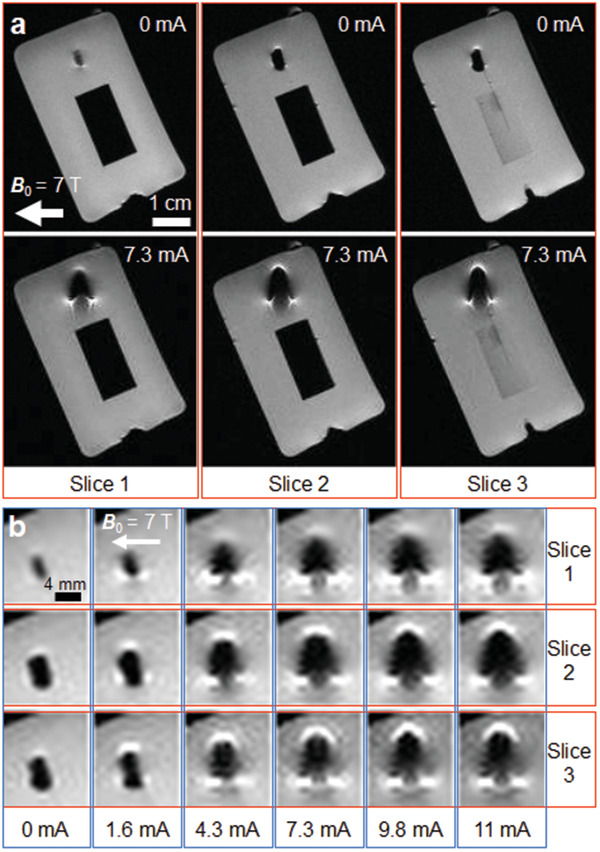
MR image artifact characterization of the single wireless actuator module in off and on states. a) Three different MR image slices of the nonoperated (off, 0 mA) actuator showing no image artifact and operated actuator showing beam bending and generated image artifacts inside preclinical MRI scanner (*B*_0_ = 7 T). b) Growing image artifacts are shown at three different image slices of the coil with increasing applied current passing through it by the application of different laser intensities.
